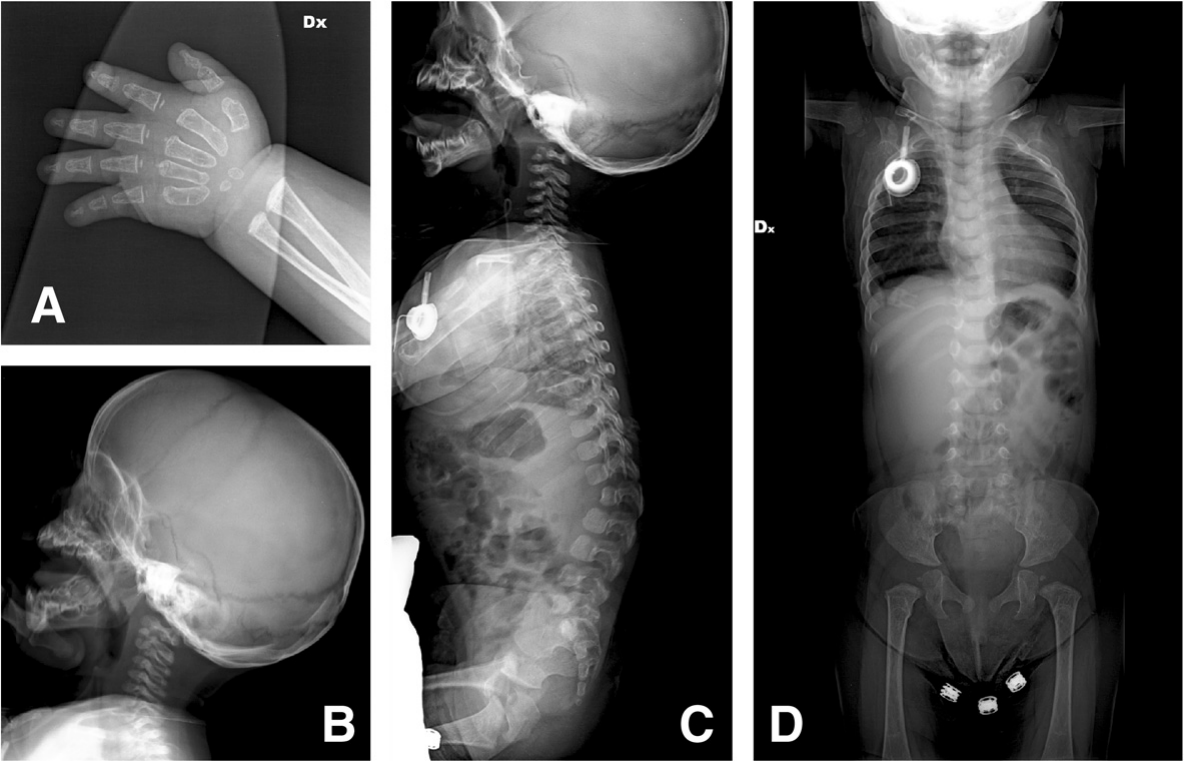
X-Rays. A: broad and hypoplastic first metacarpals, pointing of the second and third metacarpals, irregularity of the distal radial and ulnar metaphysis. B: platyspondily of the cervical vertebral bodies. C: hypoplastic vertebral body of L2, lumbar kyphosis. D: broad ribs.
